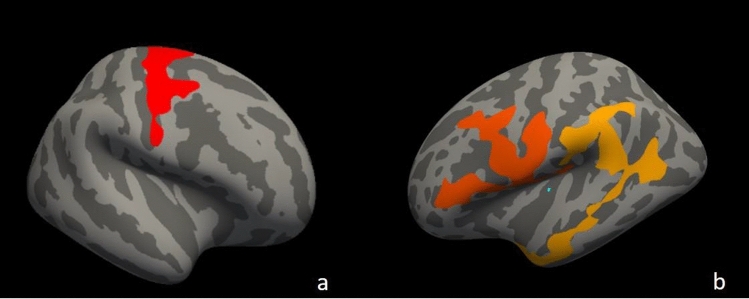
Vertex-wise analysis of CT in ALS-EDA patients related to CS. ALS-EDA revealed cortical thinning in the right precentral and paracentral cortex (a) and in the left precentral, pericalcarine and inferior-parietal cortex (b). The coloured areas represented the significance maps after Monte Carlo cluster-wise correction
